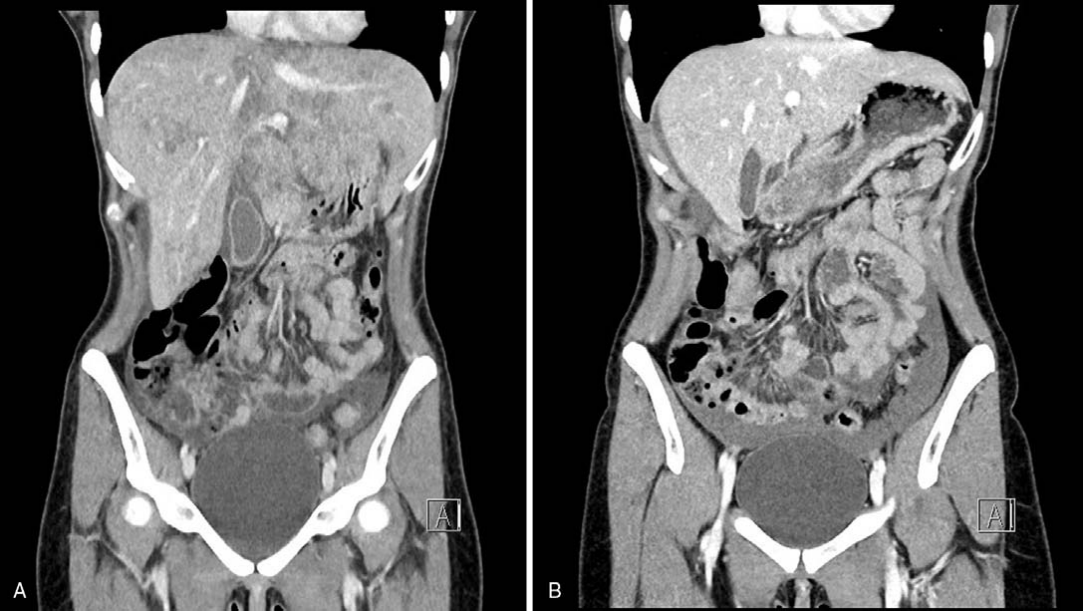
(A) Abdominal computed tomography (CT) when abdominal pain developed. Hepatosplenomegaly and edema of the gallbladder wall and liver parenchyma, hepatic hilar adenopathy, and ascites, findings suggestive of acute hepatitis were apparent. (B): Abdominal CT performed 3 weeks earlier during gynecological surgery. The liver and spleen are normal.
